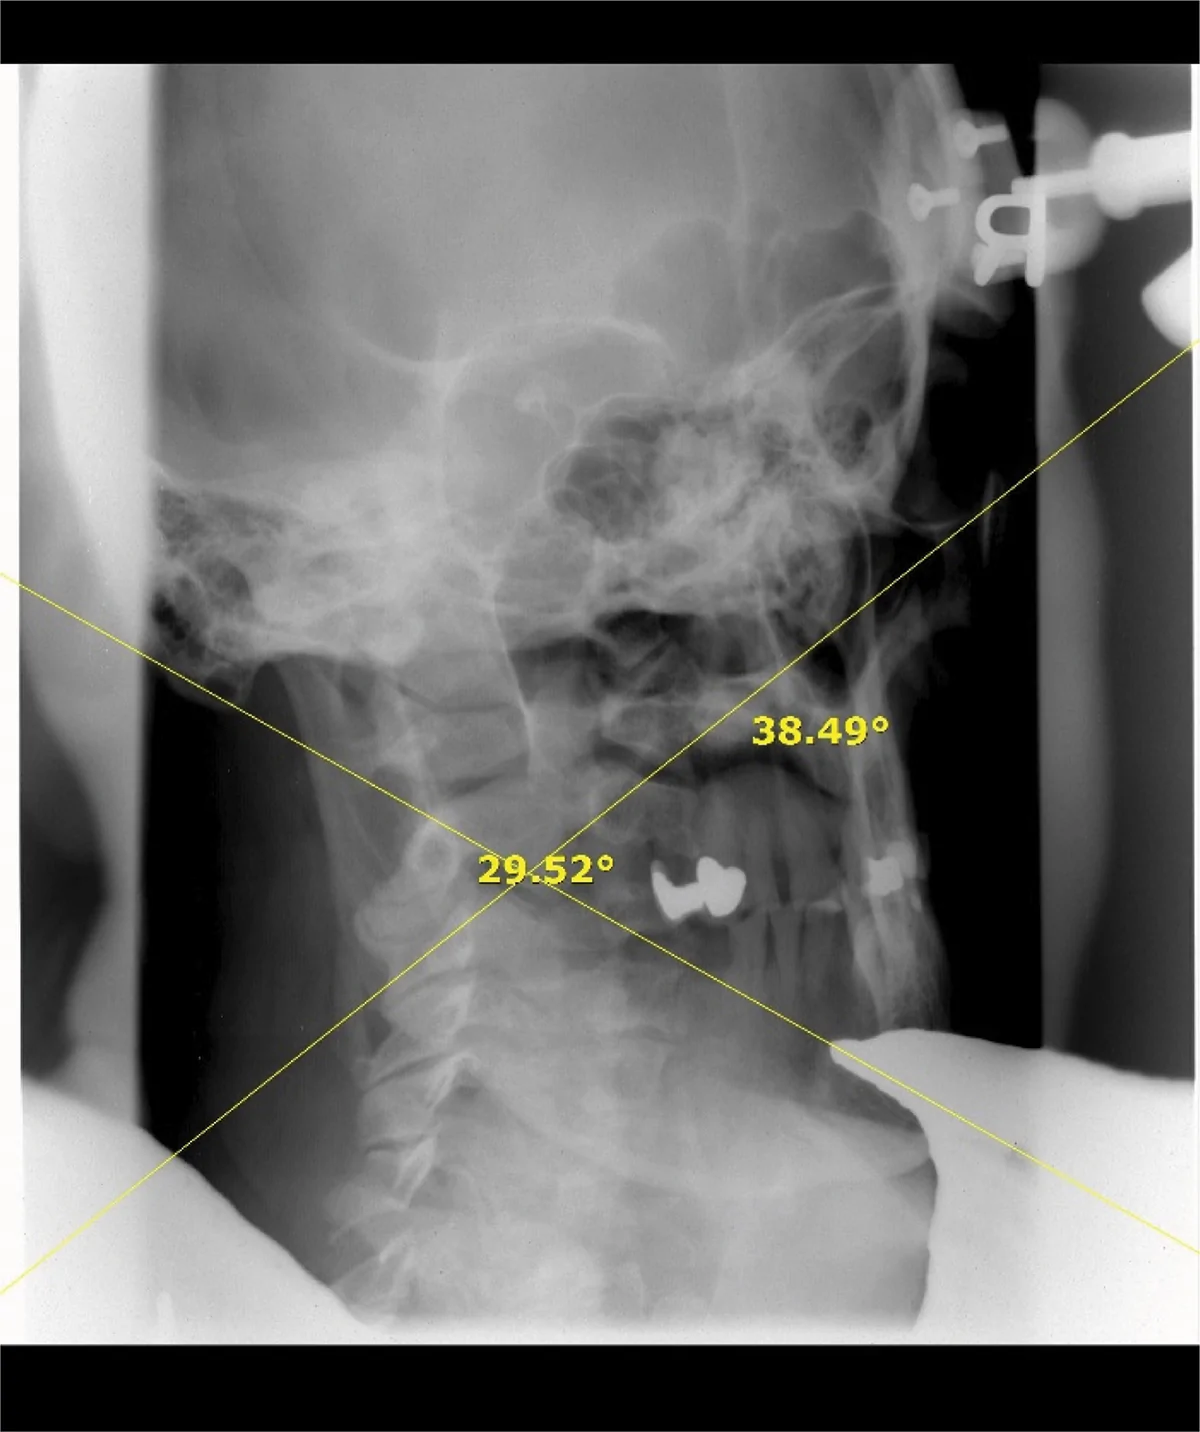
Right protracto view radiograph.
